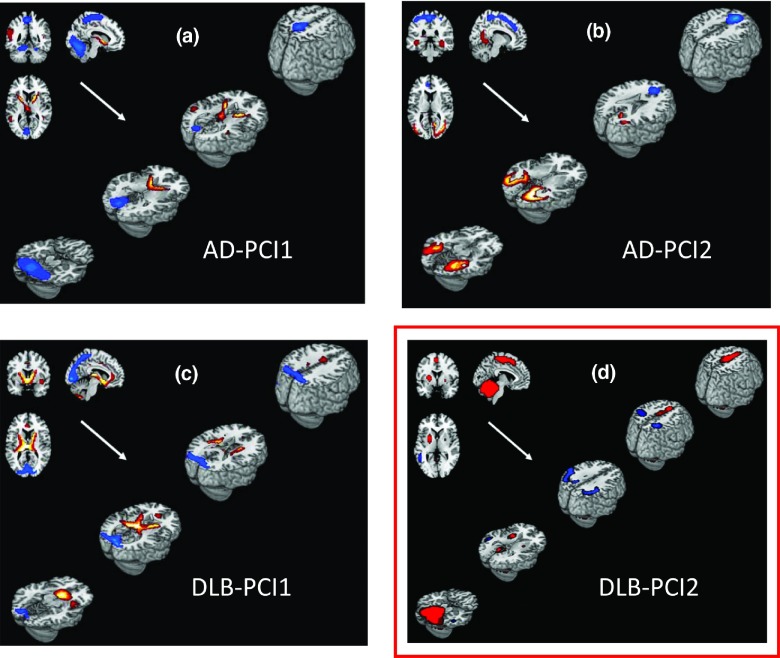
Covariant perfusion patterns in Alzheimer's disease (AD) and dementia with Lewy bodies (DLB) cohort contributing more than 10% of the variance superimposed onto structural MRI template images. Images show concomitant increase (red) and decrease (blue) regional cerebral blood flow (thresholded at |Z| ≥ 1.64, p ≤ 0.05). PCI = principal component image. Note only DLB-PCI2 correlated with clinical variables (boxed in red).
